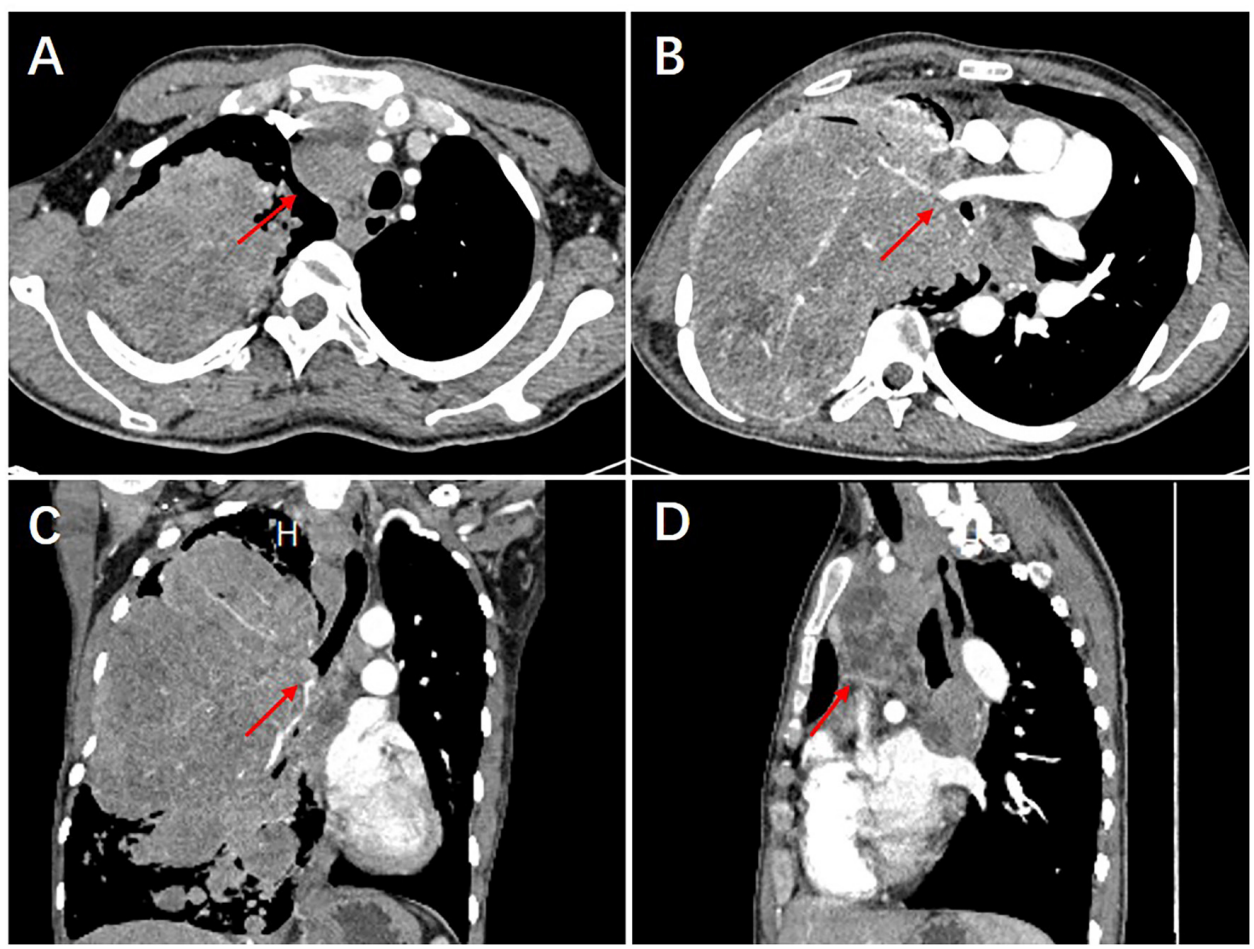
The 2019 chest CT examination showed an irregular large mass in the right lung, about 226 × 145 × 143 mm in size, with calcification and some low-density areas. The scan showed uneven enhancement, after injection of the contrast medium. Multiple nodules of varying sizes were also seen in the lungs surrounding the mass (A). The superior vena cava, some branches of the right pulmonary artery and the right pulmonary vein were also all invaded (B). In addition, bronchial stenosis in the upper, middle, and lower lobes of the right lung was observed (C). The mediastinum was shifted to the left due to compression and multiple enlarged lymph nodes were seen in the mediastinum (D).
